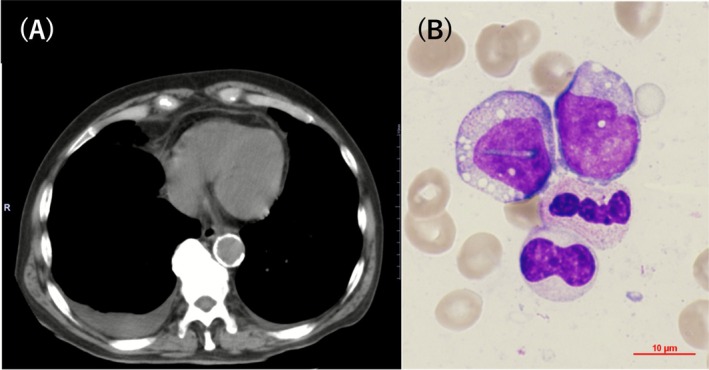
Images at the time of admission. Chest CT on admission revealed right‐predominant pleural effusion (A). Bone marrow examination showed cytoplasmic vacuoles in myeloid precursors (B).
